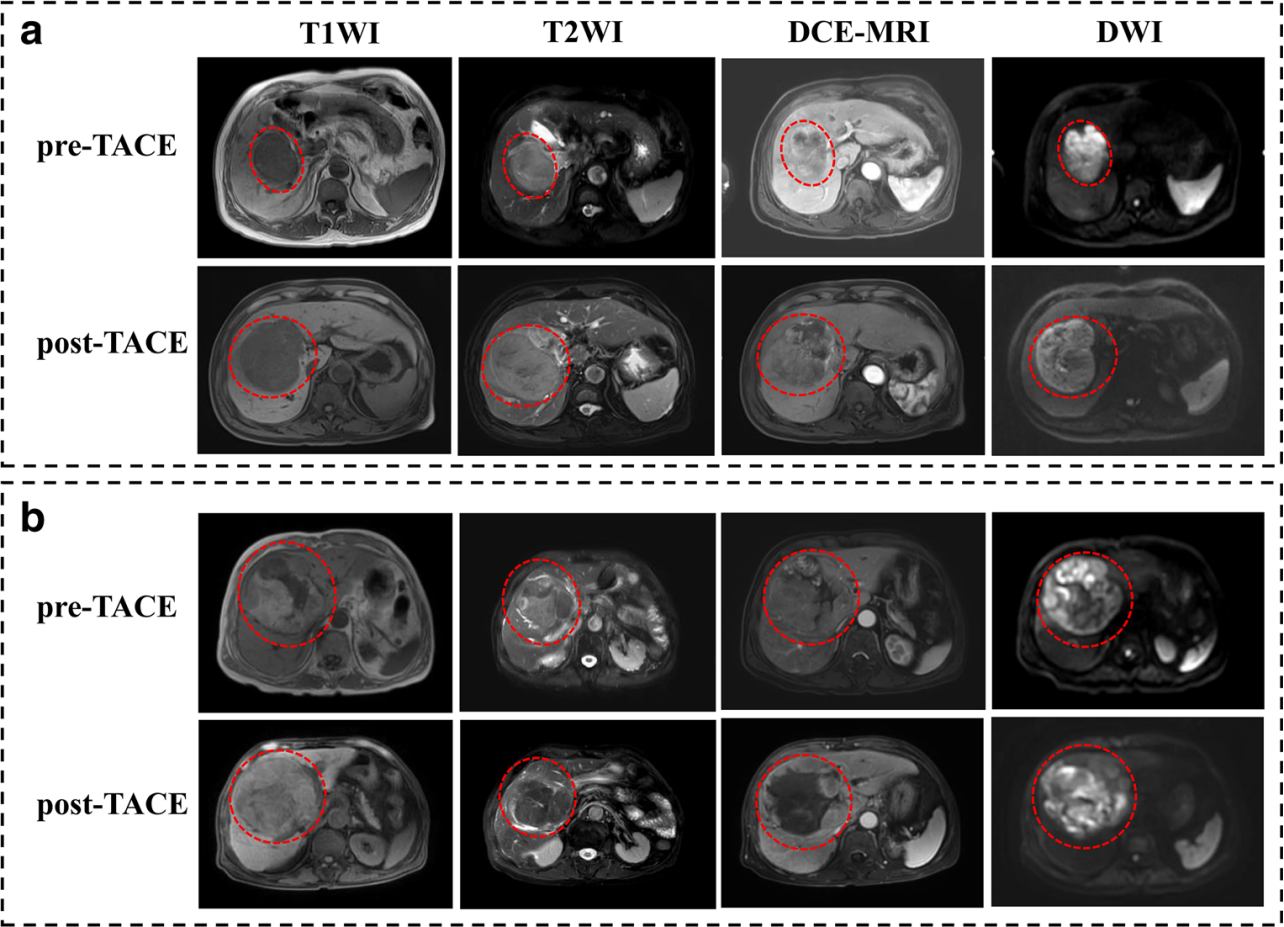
Representative MR images for nTR and TR of HCC patients according to mRECIST criterion. a A 73-year-old male HCC patient with a lesion diameter of 69.82 mm underwent MR scanning 1 week before TACE, followed by MR scanning 42 days after TACE. T1WI, T2WI, DCE-MRI, and DWI images were collected, and the results showed that the tumor presented with nTR, with the lesion diameter increasing to 98.79 mm. b An 80-year-old male HCC patient, with a lesion diameter of 69.20 mm. MR scanning was performed 1 week before TACE, followed by MR scanning 42 days after TACE, and the results showed the lesion presented with partial response (TR), with the lesion diameter decreasing to 64.32 mm and 85% necrosis. MR, magnetic resonance; TACE, transcatheter arterial chemoembolization; TR, TACE response; nTR, non-TACE response; HCC, hepatocellular carcinoma; mRECIST, modified Response Evaluation Criteria in Solid Tumors
